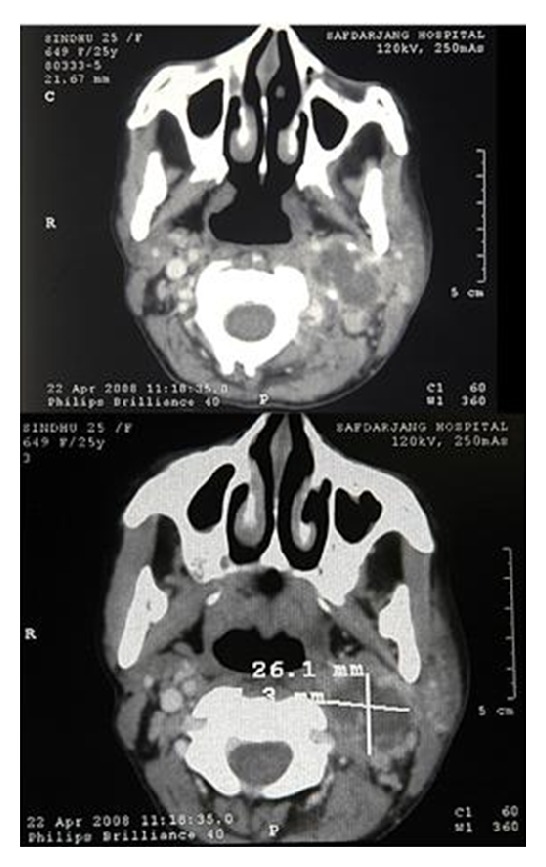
CT scan showing IJV thrombosis with carotid abscess.
